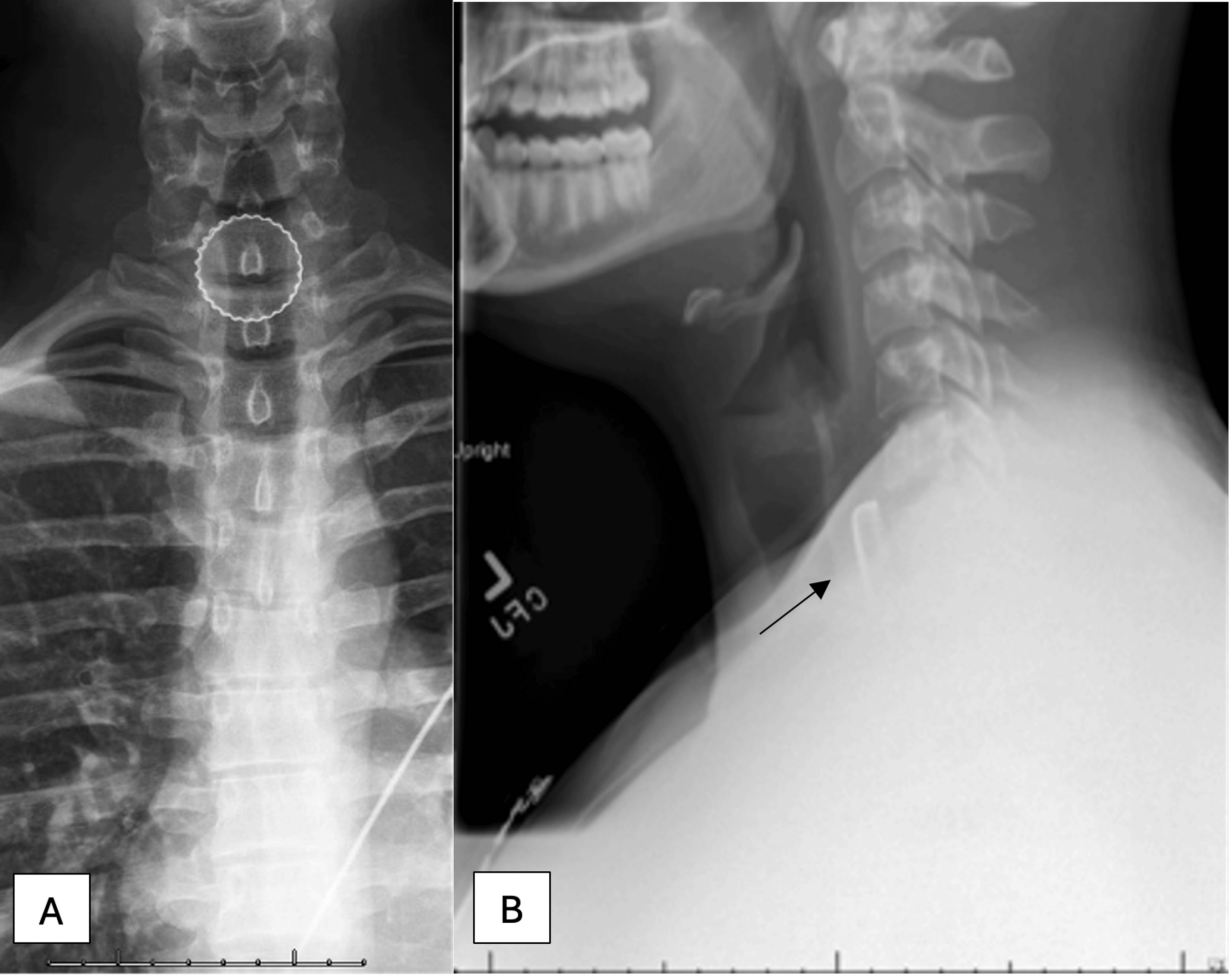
(A) Anterior-posterior and (B) lateral neck X-ray with a radiopaque foreign body at the esophageal inlet.
